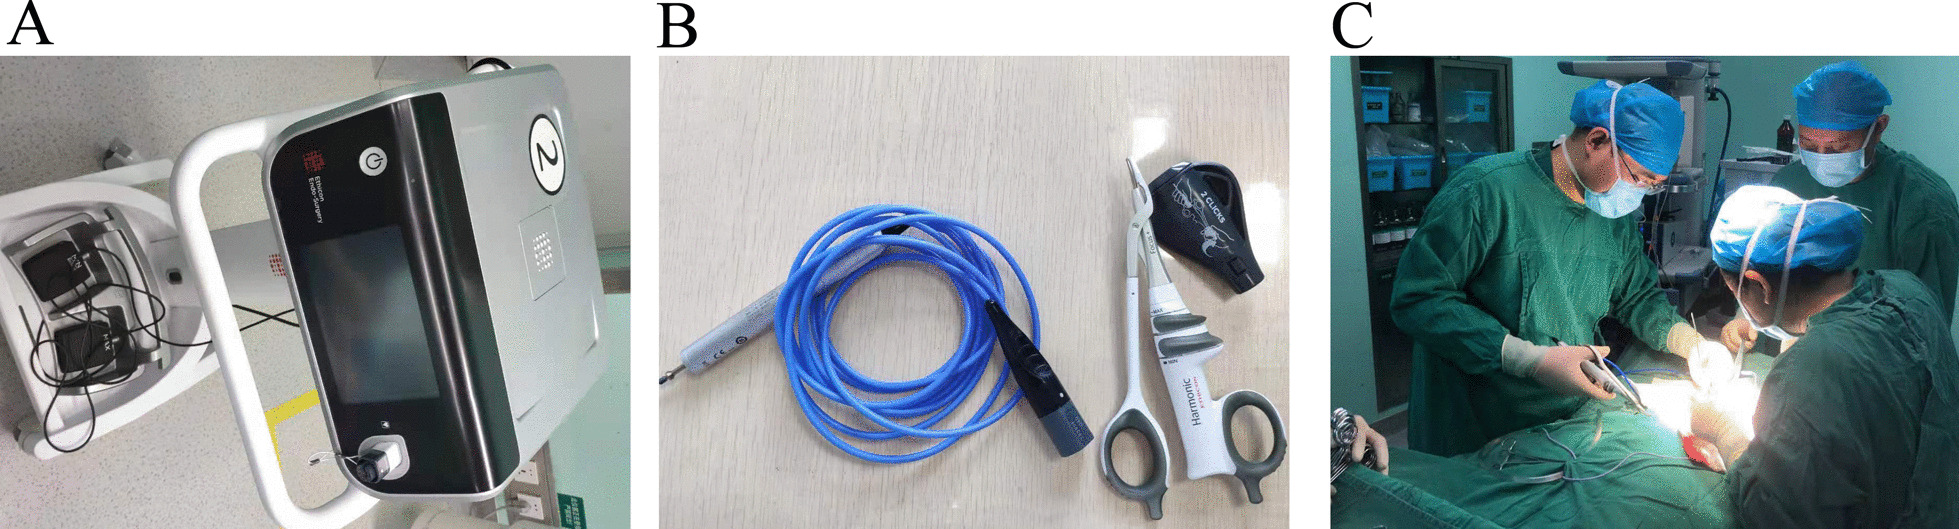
Ultrasonic scalpel is used in PTX. A and B Ultrasonic scalpel device, C Ultrasonic scalpel was used in operation
